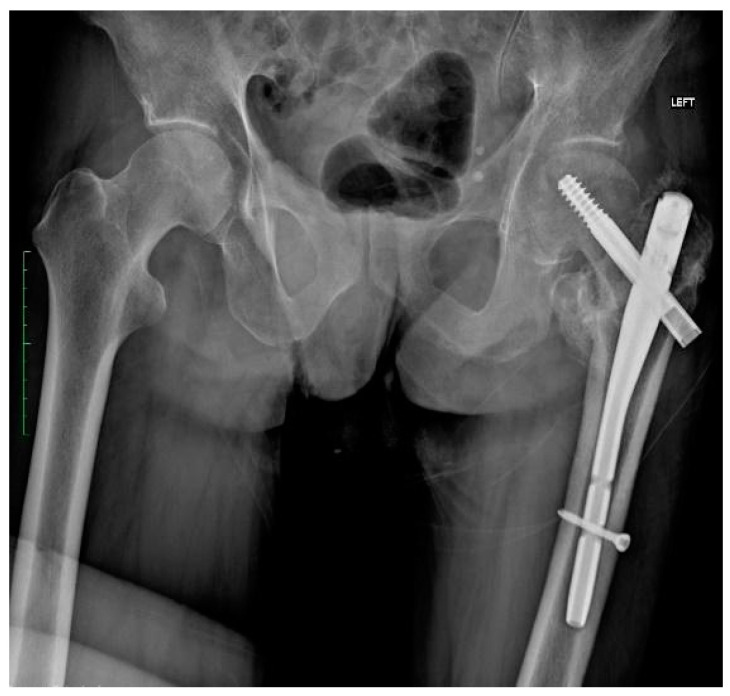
X-ray after surgery. Pertrochanteric fracture of the left lower limb fusion (Gamma nail). Left limb adduction as the result of increased tension of the spastic adductor muscles. Anterior–posterior (AP) view.
